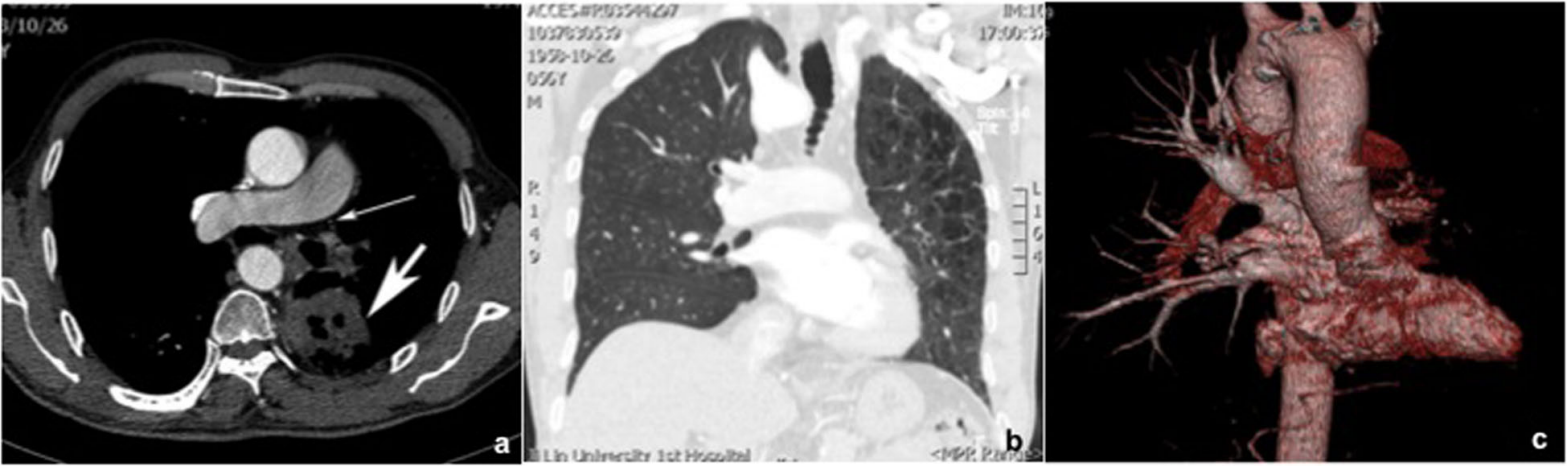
Preoperative chest CTA of a 56-year-old man showed unilateral absence of left pulmonary artery and pulmonary mass in left lower lobe. Axial contrast-enhanced CT showed a cavitary lesion measuring 5.5 cm × 5.7 cm in the superior segment of left lower lobe and absence of left pulmonary artery in its expected course (a). Coronal CT reconstructed image demonstrated the absence of left pulmonary artery and extensive emphysematous changes in the pulmonary parenchyma (b). Volume rendering CT revealed the absence of the left pulmonary arterial vasculature(c)
